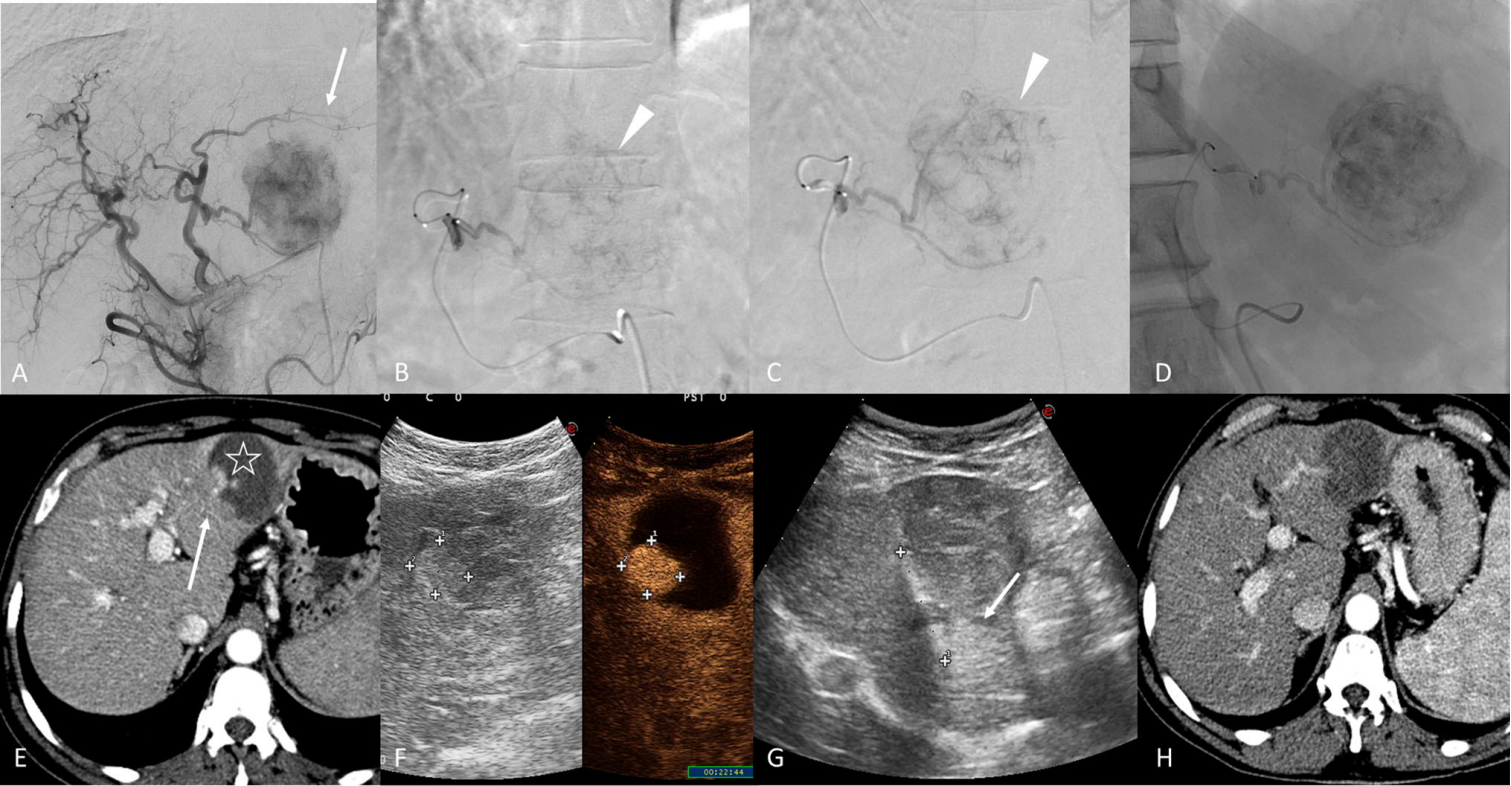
A 156-year-old male with a single nodule of HCC with maximal diameter of 76 mm at II/III hepatic segments. Figure A, Digital subtraction angiography (DSA) performed from the common hepatic artery, shows the hypervascular structure of the HCC in the left lobe (arrow). Super-selective DSA of the tumour with deflated balloon figure B) and inflated balloon figure C) (arrowhead). Figure D shows single fluoroscopy image after the embolization. Figure E, 1-month follow-up CT in arterial phase, highlights the partial necrosis of the nodule (star) with the presence of a hypervascular bottom (arrow) of vital residual tumour. Figure F, contrast-enhanced ultra-sound, shows the residual HCC, and figure G evidences the radiofrequency ablation of the lesion (arrow). Figure H, post-procedural CT in arterial phase, evidences the complete response of the HCC
